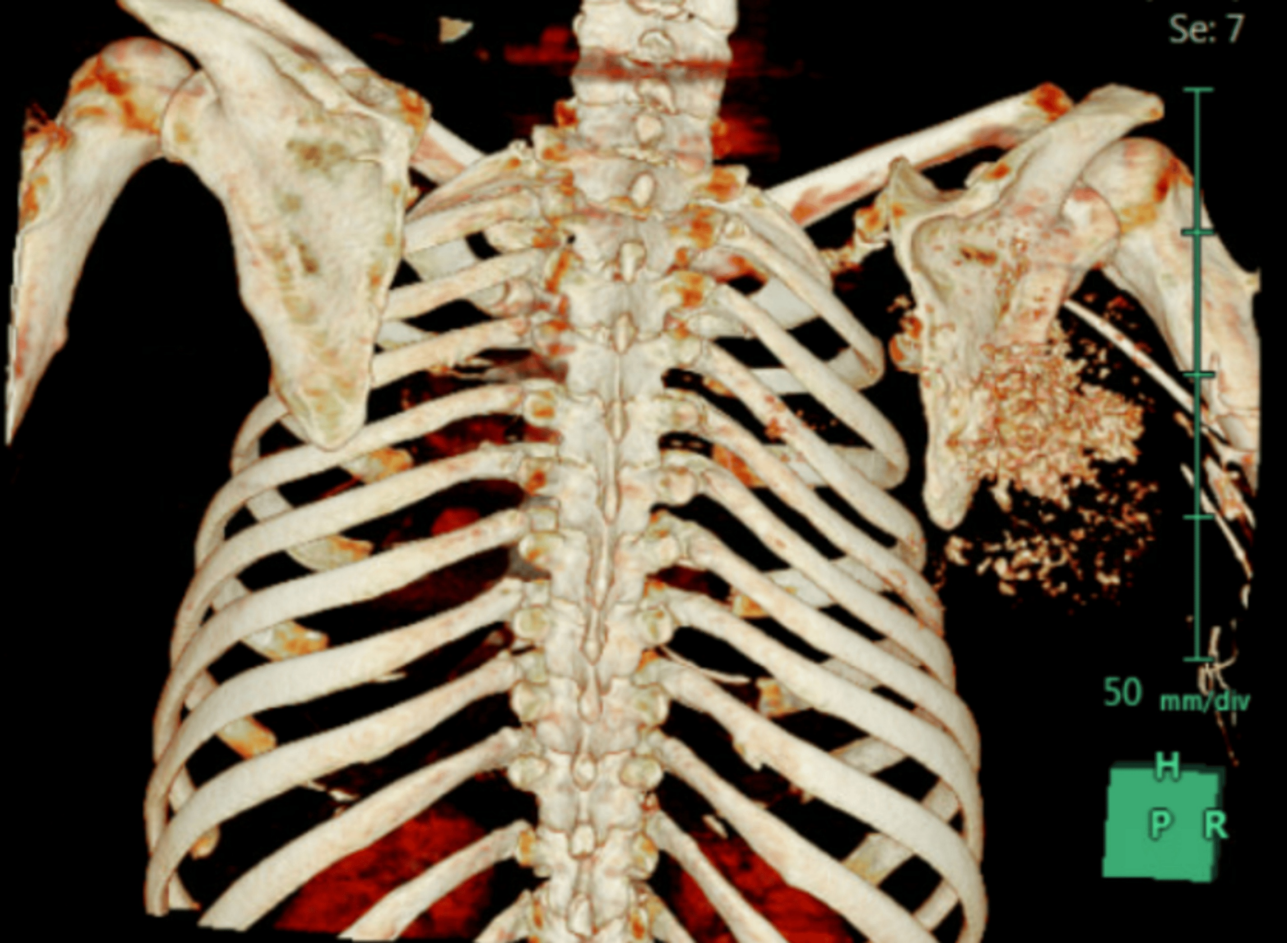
Preoperative 3D CT scan image of the scapula showing the extensive destruction of the lower two-thirds of the scapular body.
